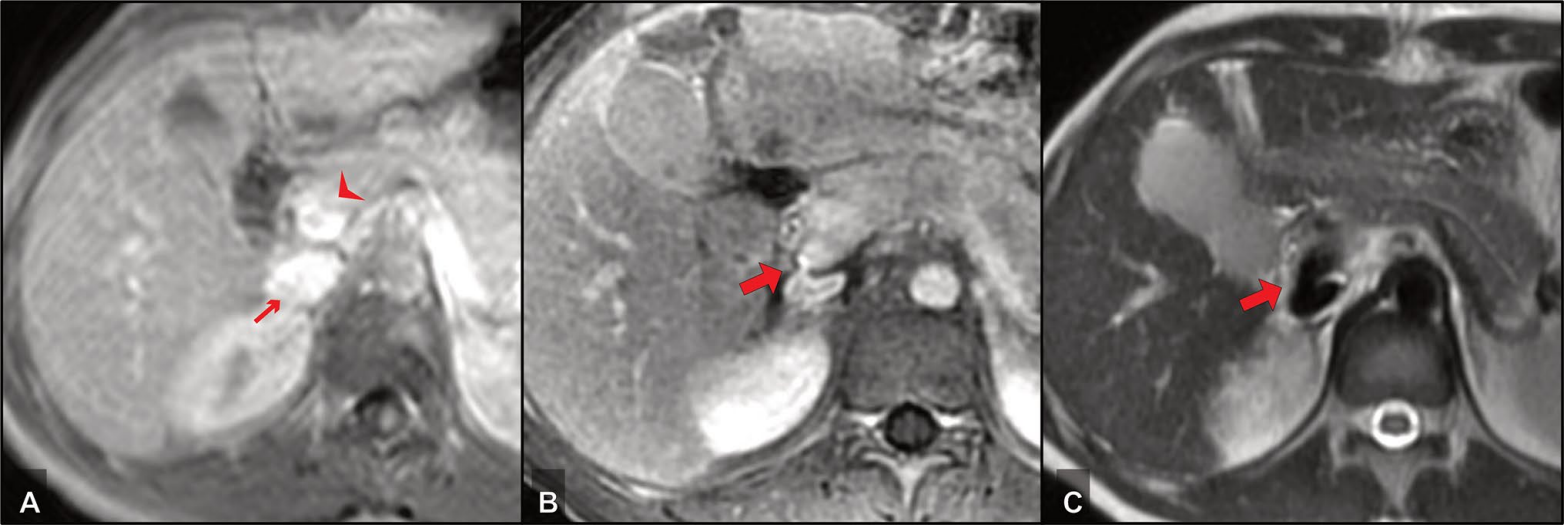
A 17-year-old female PHT patient with Wilson’s disease and recurrent variceal bleeding—PSSS procedure: portacaval side-to-side; a preoperative MRI, post-contrast T1-w, b postoperative MRI, post-contrast T1-w and c postoperative MRI, T2w. Small arrow: IVC; arrowhead: portal vein; bold arrow: portacaval anastomosis
